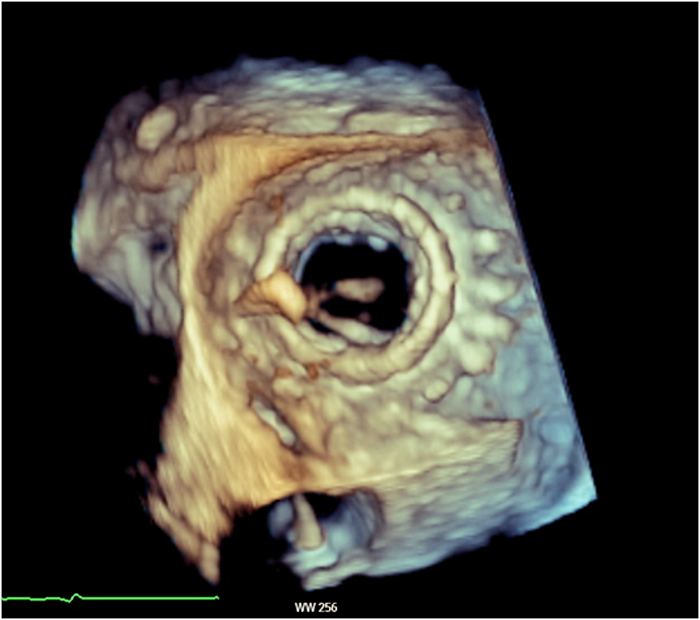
3-Dimensional Transesophageal Echocardiography of the Mitral Valve Prosthesis After Deployment Three-dimensional transesophageal echocardiography of the mitral valve after deployment of the balloon-expandable valve demonstrates an excellent result and no paravalvular leak.
